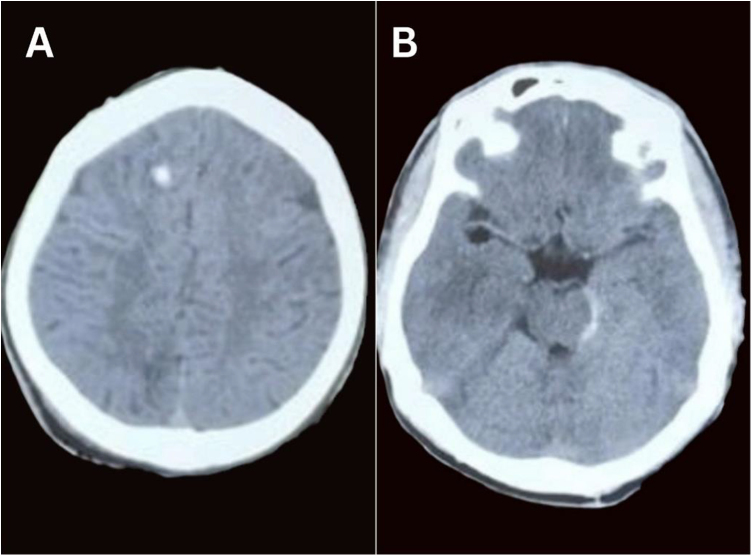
CT head showing hyperdense lesion in the right frontal region and subarachnoid hemorrhage over left peri mesencephalic cistern.
